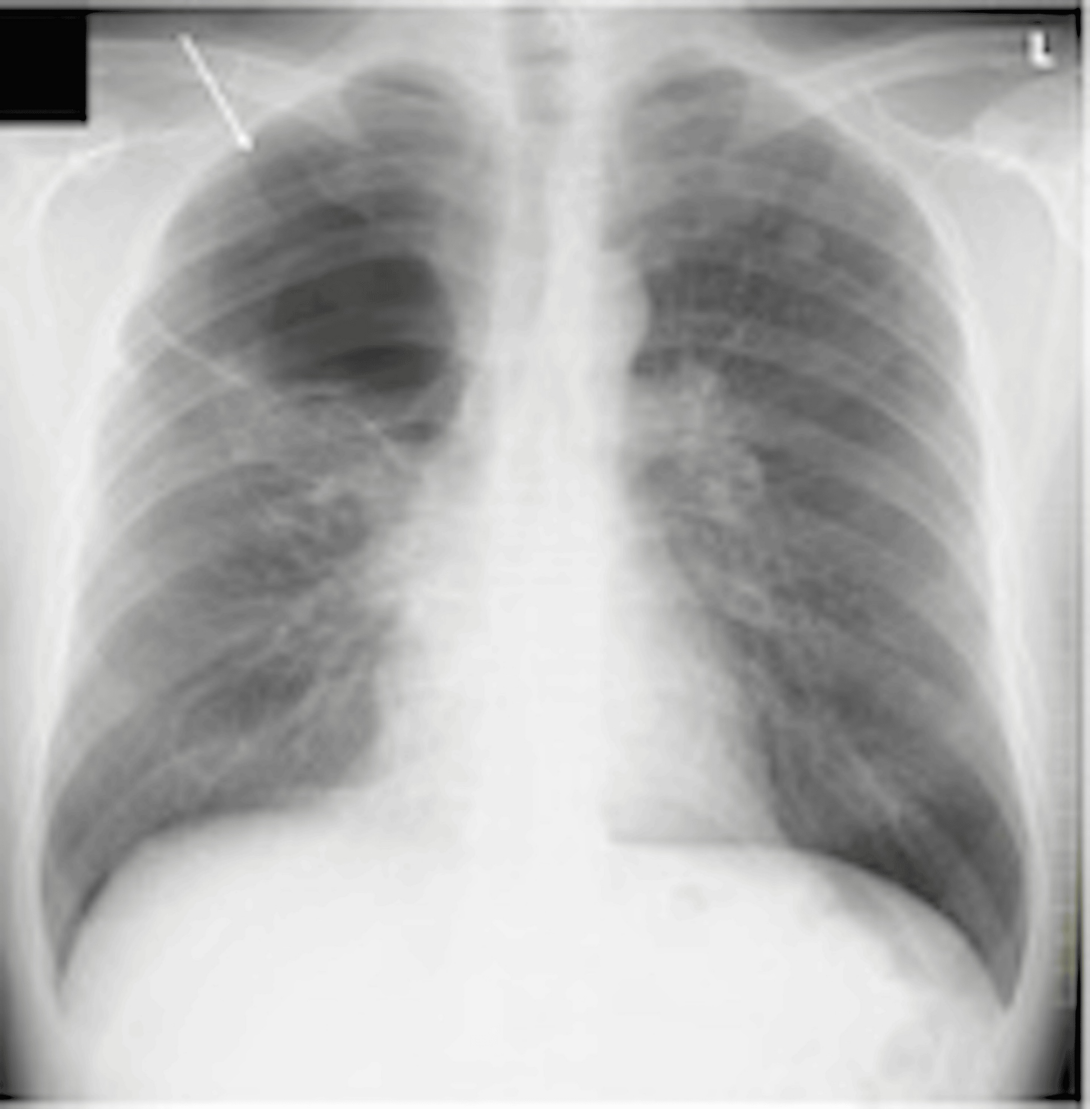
Chest radiograph (frontal view). Cystic lesion in the apex of the right lung.
